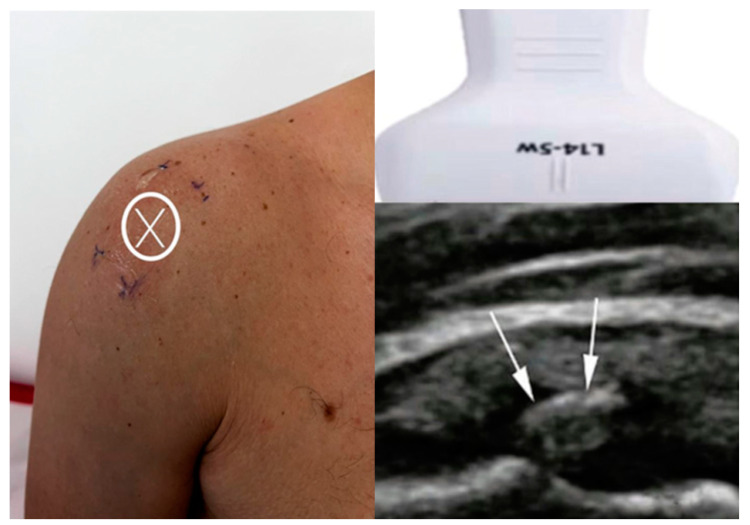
Ultrasound-guided targeting in Group 1. The X mark indicates the skin projection of the calcific deposit, and the arrows highlight the deposit on ultrasound imaging.
